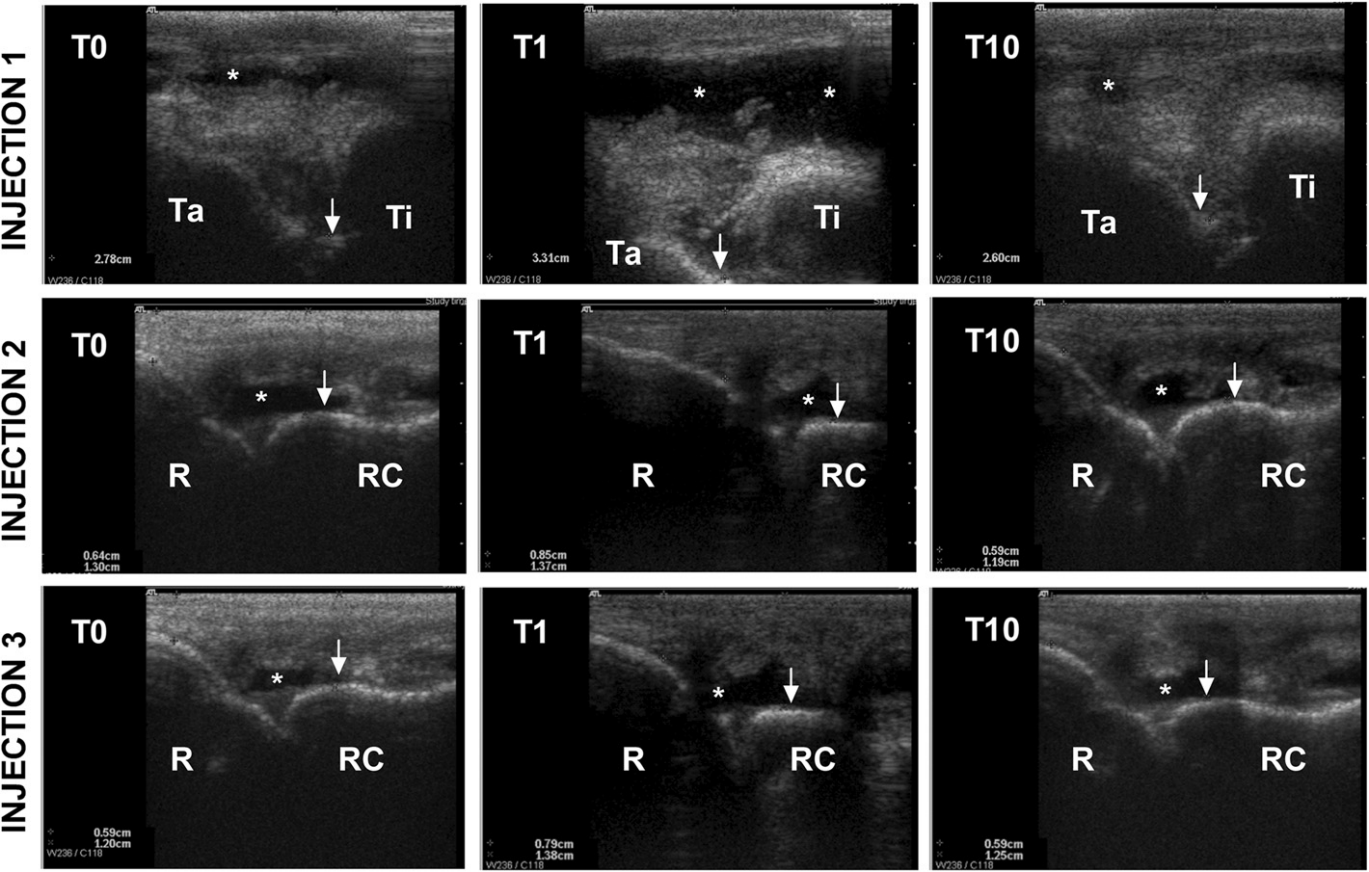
Longitudinal ultrasound images of representative MSC-injected tarso-crural and radio-carpal joints after Injections 1, 2 and 3. The transducer was longitudinally placed on the lateral plantar pouch of the tibiotarsal joint or in the dorsal aspect of the carpus, parallel and medial to the extensor carpi radialis tendon, at the level of the radio-carpal joint. Images are recorded previous to the IA injection of BM-MSCs (T0), and 1 day (T1) and 10 days (T10) after BM-MSC injection. Distance between the bone surface and the skin was measured in two points at every time. Slight distension and effusion was observed 1 day after all BM-MSC injection, which was normalized by the 10^th^ day. Ta = talus, Ti = tibia, R = radius, RC = radio-carpal bone, * = synovial fluid (effusion) in tarso-crural or radio-carpal joint, white arrow indicates the bone surface
